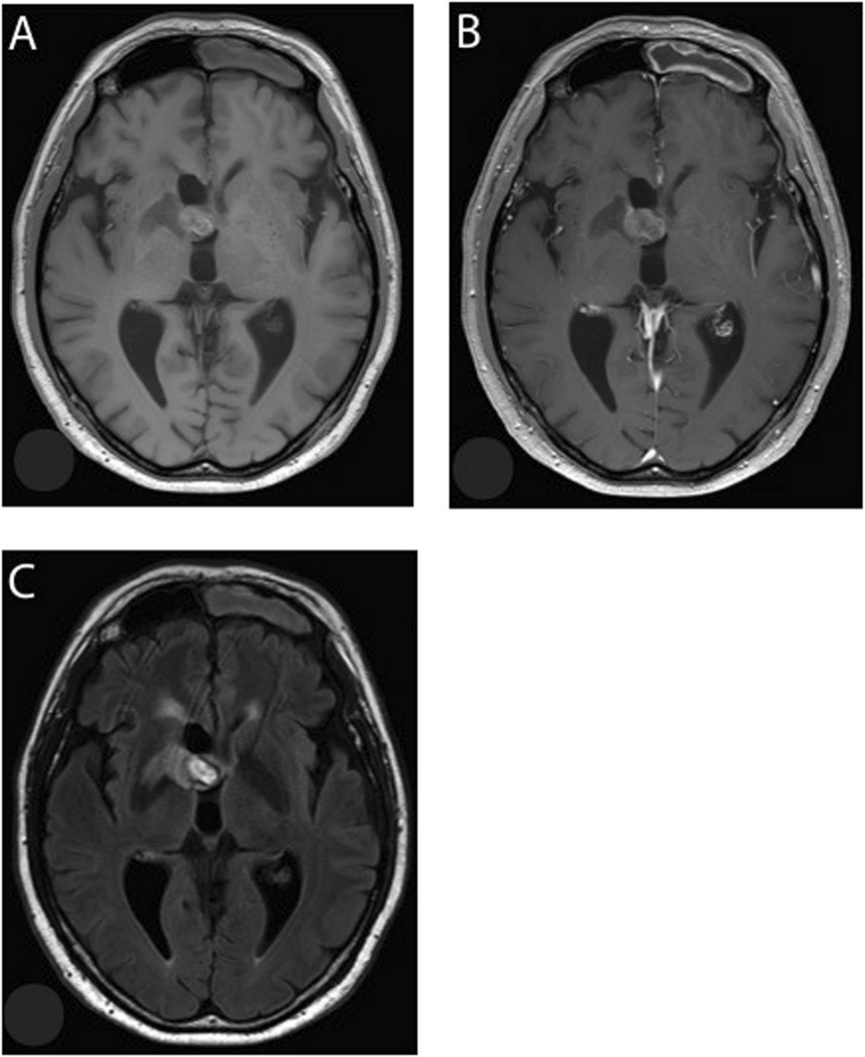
Treatment response after 17 courses of bevacizumab in cMRI. A Non-enhanced T1-weighted MRI reveals further decrease in lesion size. Hyperintense hemorrhagic changes are present within the medial portion of the tumor. B Gadolinium-enhanced T1-weighted MRI documents lack of contrast enhancement in the lateral portions of the tumor. Only a slight enhancement in addition to the hyperintense hemorrhagic changes in the medial portion of the tumor was observed. C T2-weighted FLAIR demonstrates a decrease of peritumoral edema.
